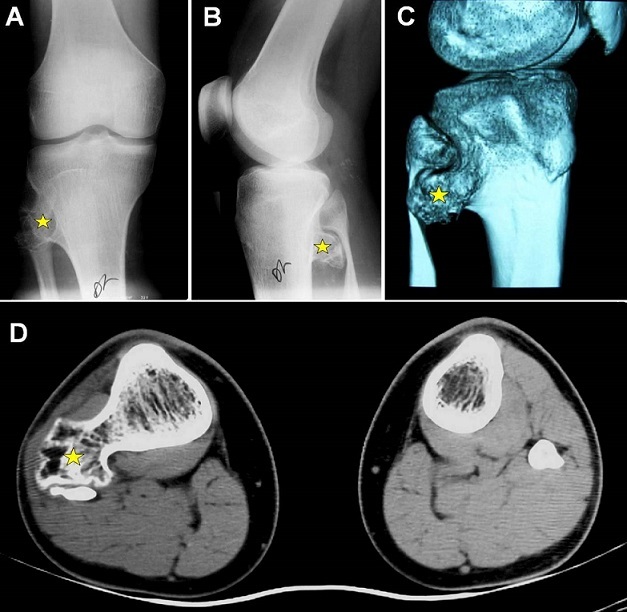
Right knee: Antero-posterior (A) and lateral (B) plain radiographs. 3-D CT-scan bony reconstruction (C) with axial CT-scan of both proximal tibias and fibulas (C-D) showing a large osteophytic bony lesion (star) in the proximal metaphyse of the right tibia with an important mass effect on the fibular head which is deformed (A-D)
